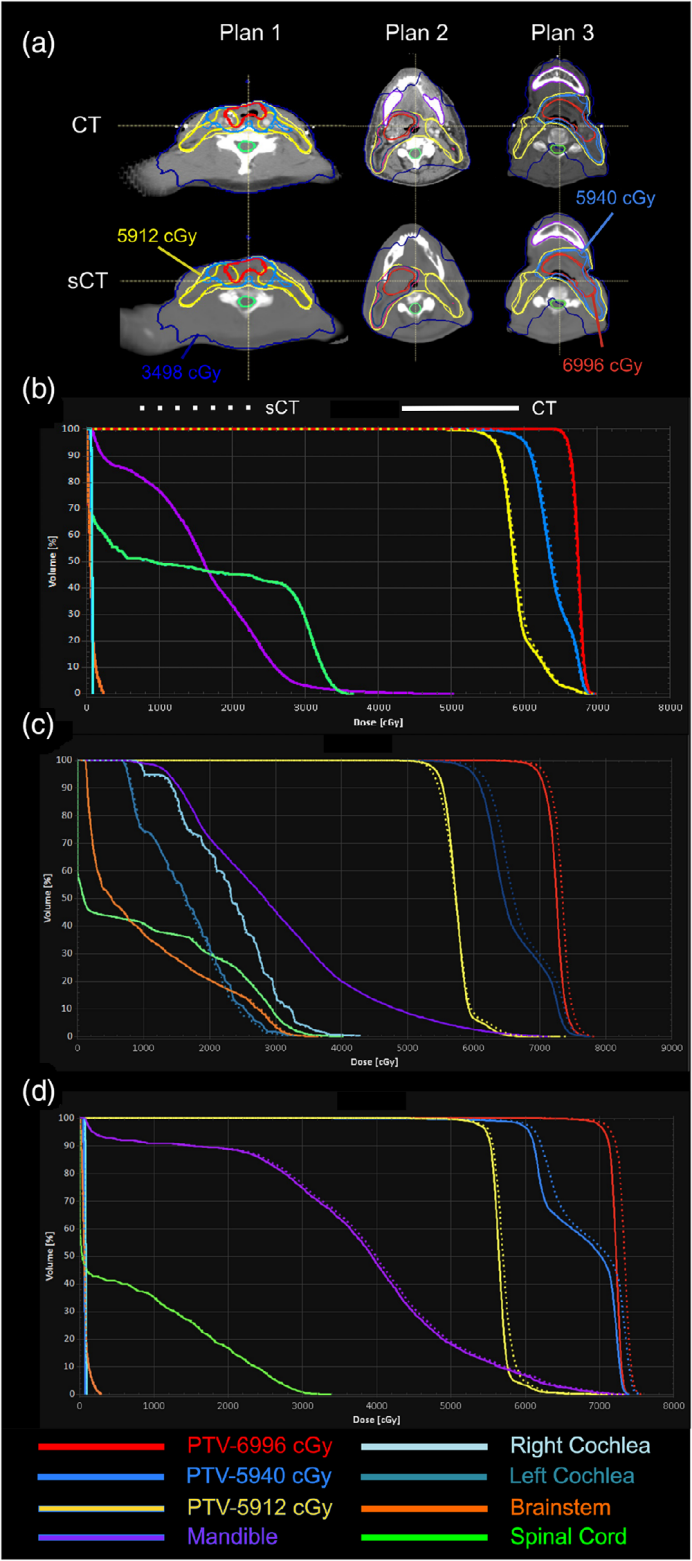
(a) Axial slice showing a CT computed treatment plan recalculated on sCT for plans with a modulation factor = 3 (Plan 1), modulation factor = 4 (Plan 2) and modulation factor = 5 (Plan 3). The D20 (720 cGy), 5412 cGy, 5940 cGy, and 6996 cGy isodose lines are shown (dark blue, yellow, light blue, red) overlaid with the 5412 cGy (yellow), 5940 cGy (light blue) and 6996 cGy (red) PTV structures. DVH for CT (solid lines) and sCT calculated plans (dashed lines) are shown for Plan 1 (b), Plan 2 (c), and Plan 3 (d).
